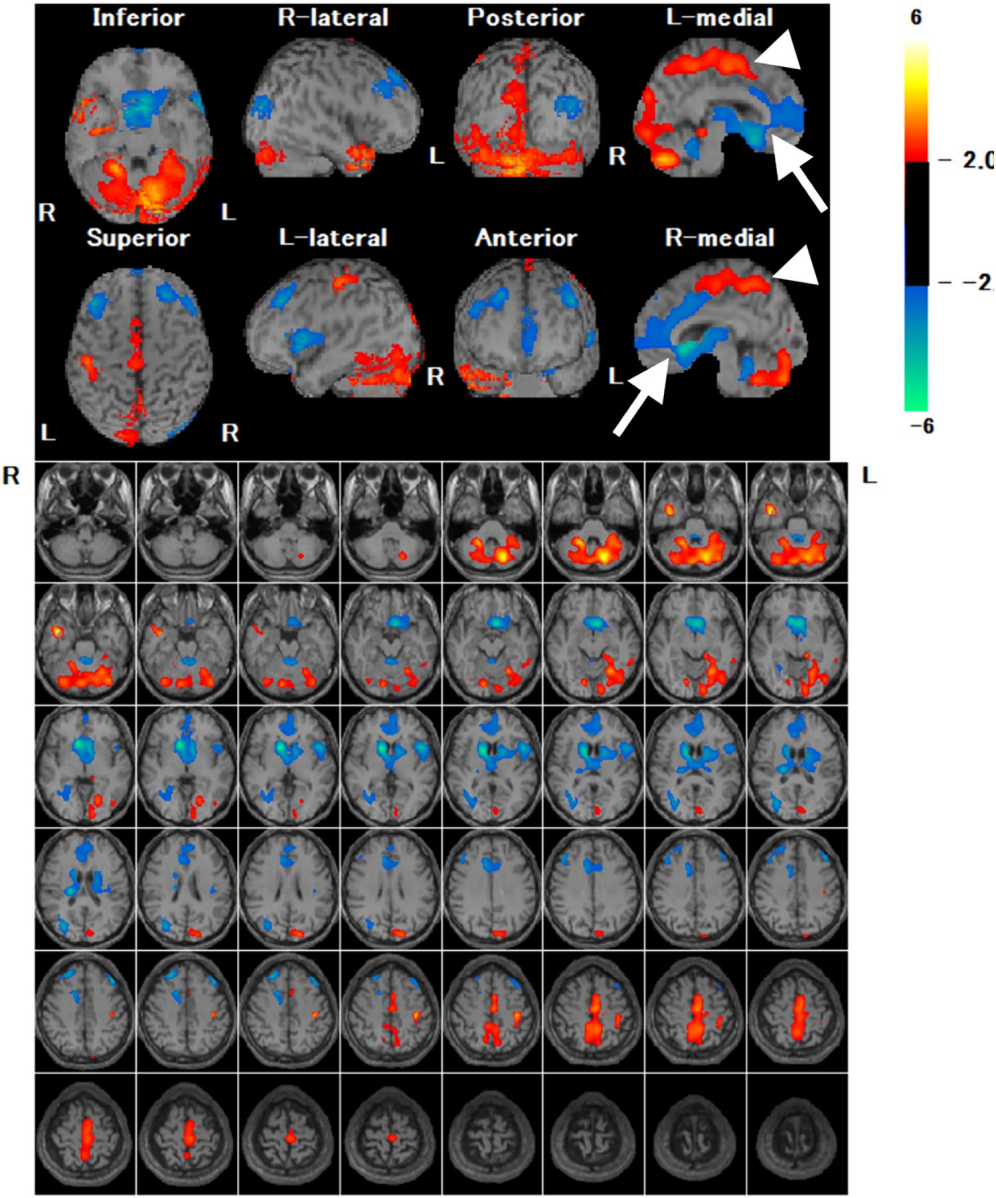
A representative case of iNPH. eZIS analysis of ECD brain perfusion SPECT images showed CAPPAH sign (arrowheads) and apparent hypoperfusion around the ventricles (arrows)
